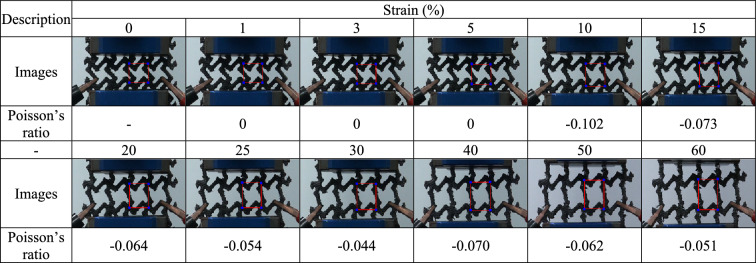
Strain images and Poisson’s ratio of auxetic lattice CT patterns up to 60% elongation.
